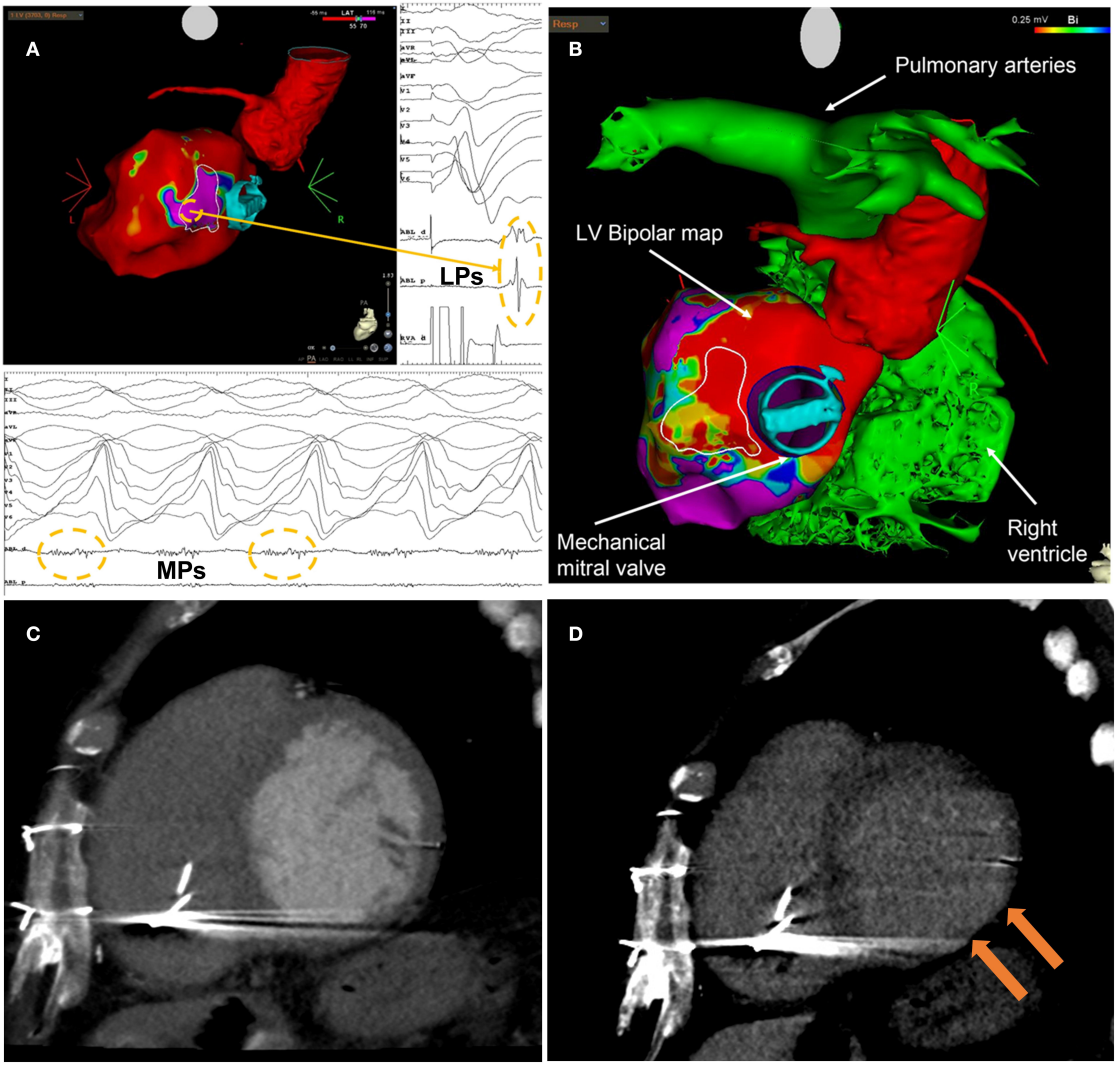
(A) Endocardial electroanatomic substrate map (CARTO3, Biosense Webster, Irvine, CA, USA) obtained from the second VT ablation procedure. The map highlights the area of late potentials (LPs) characterized by a local late activation time (LAT) after paced QRS end, located at the basal inferolateral segment of the left ventricle. Mesodiastolic potentials (MPs) recorded during the clinical VT were located at the same spot of the farthest LPs. (B) Integration of CT imaging with CARTO imaging data (bipolar voltage map). In both (A,B), the white outlined area is the arrhythmogenic target for STAR identified at EAM mapping. (C) LV short axis view of cardiac CT angiographic phase with thinned basal inferolateral myocardium. (D) LV short axis view of late cardiac CT phase with hyperdensity on basal inferior-lateral wall that represents transmural fibrosis.
